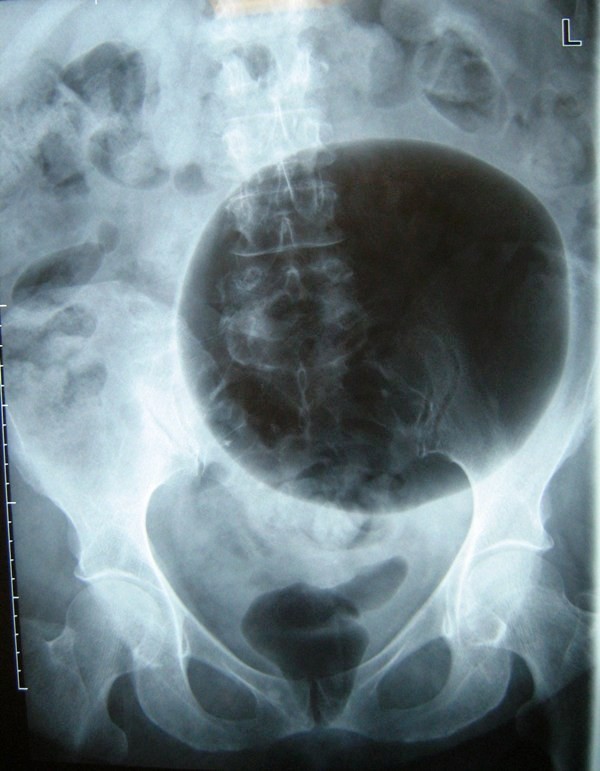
An abdominal X-ray showing air filled giant diverticulum.
